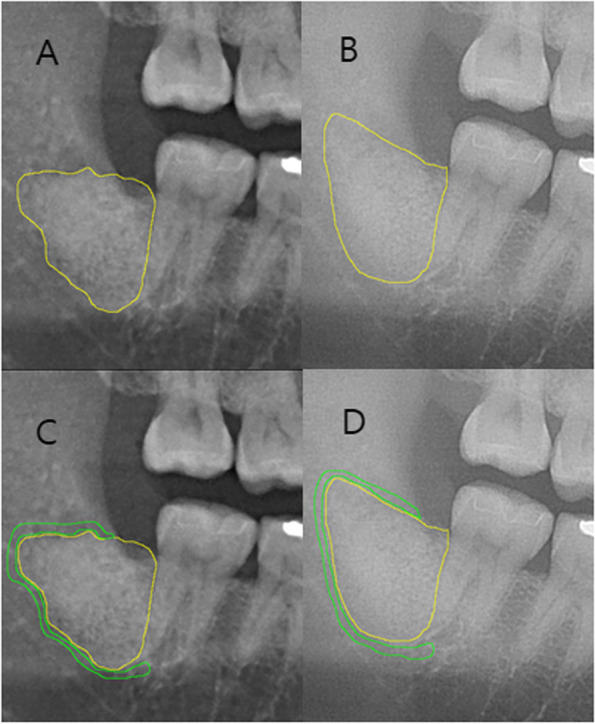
Method for measuring bone density using panoramic radiographs. A Postoperation 1st visit defect region. B Postoperation 2nd visit defect region. C Postoperation 1st visit surrounding bone. D Postoperation 2nd visit surrounding bone
